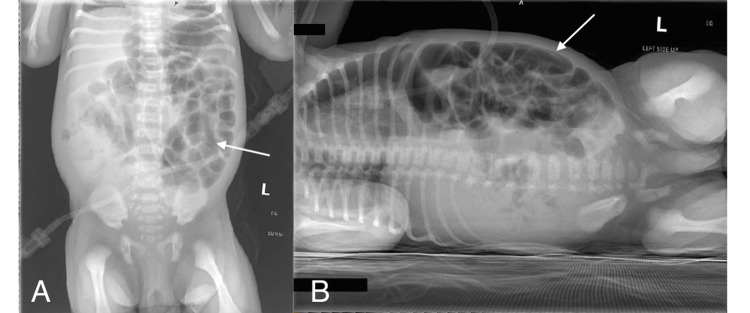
X-ray abdomen taken at first admission (A) *Supine positioning.* The arrow points to multiple air-filled small bowel loops; (B) *Right lateral decubitus positioning.* The arrow points to multiple air-filled small bowel loops.
